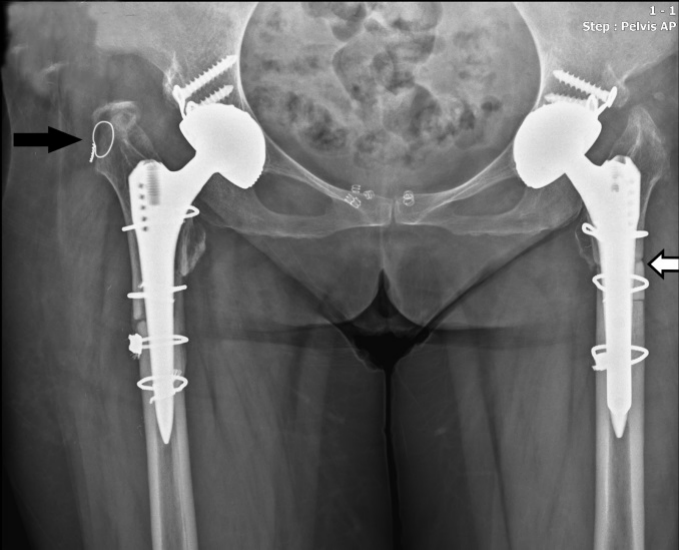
X-ray radiography of patient whose trochanter major fracture (black arrow) was repaired with cerclage with a fracture that developed in the intraoperative osteotomy line (white arrow).
